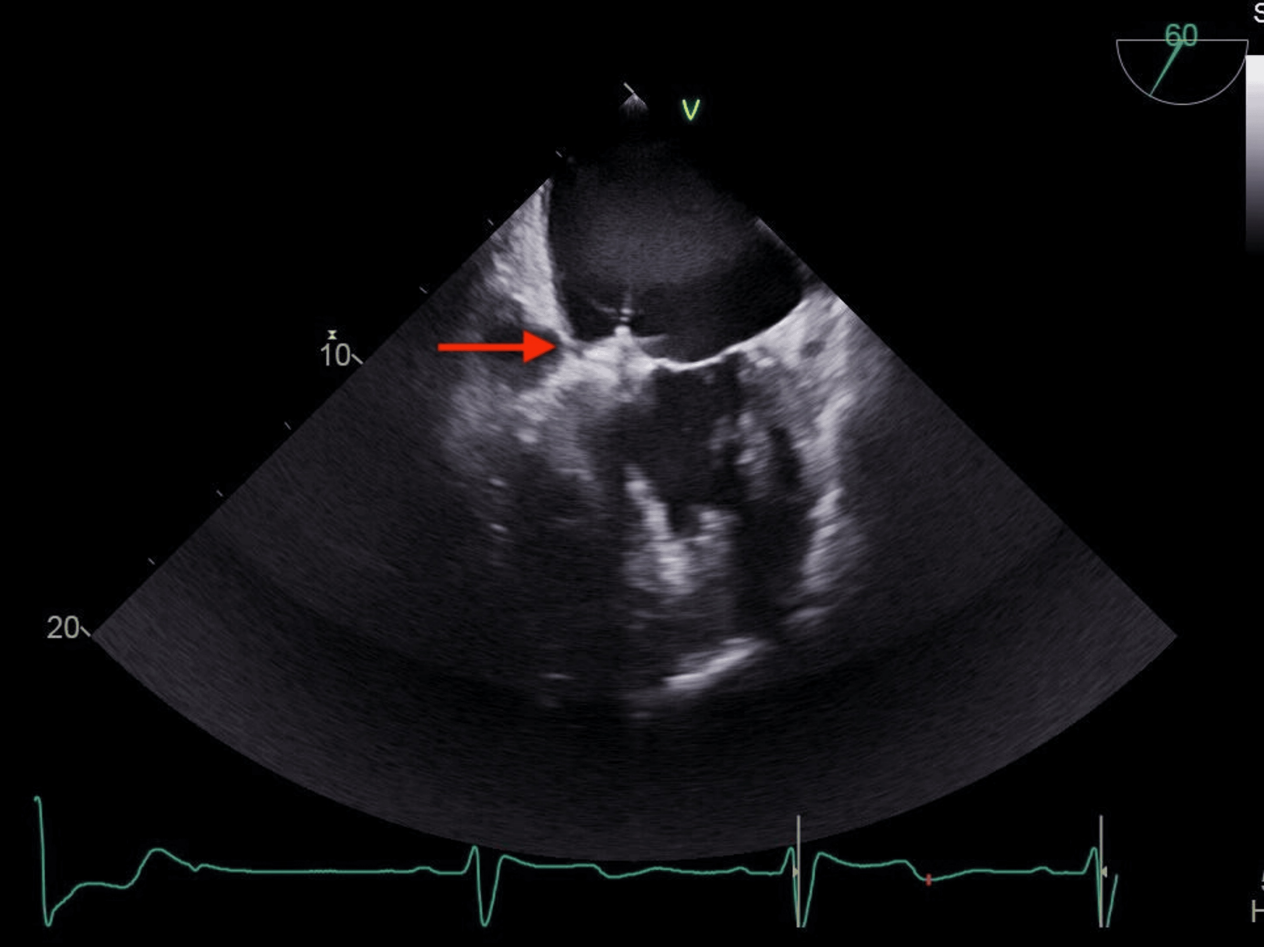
Transesophageal view of the mitral valve at 60°. The Amplatzer device is appropriately seated across the mitral valve (red arrow)
